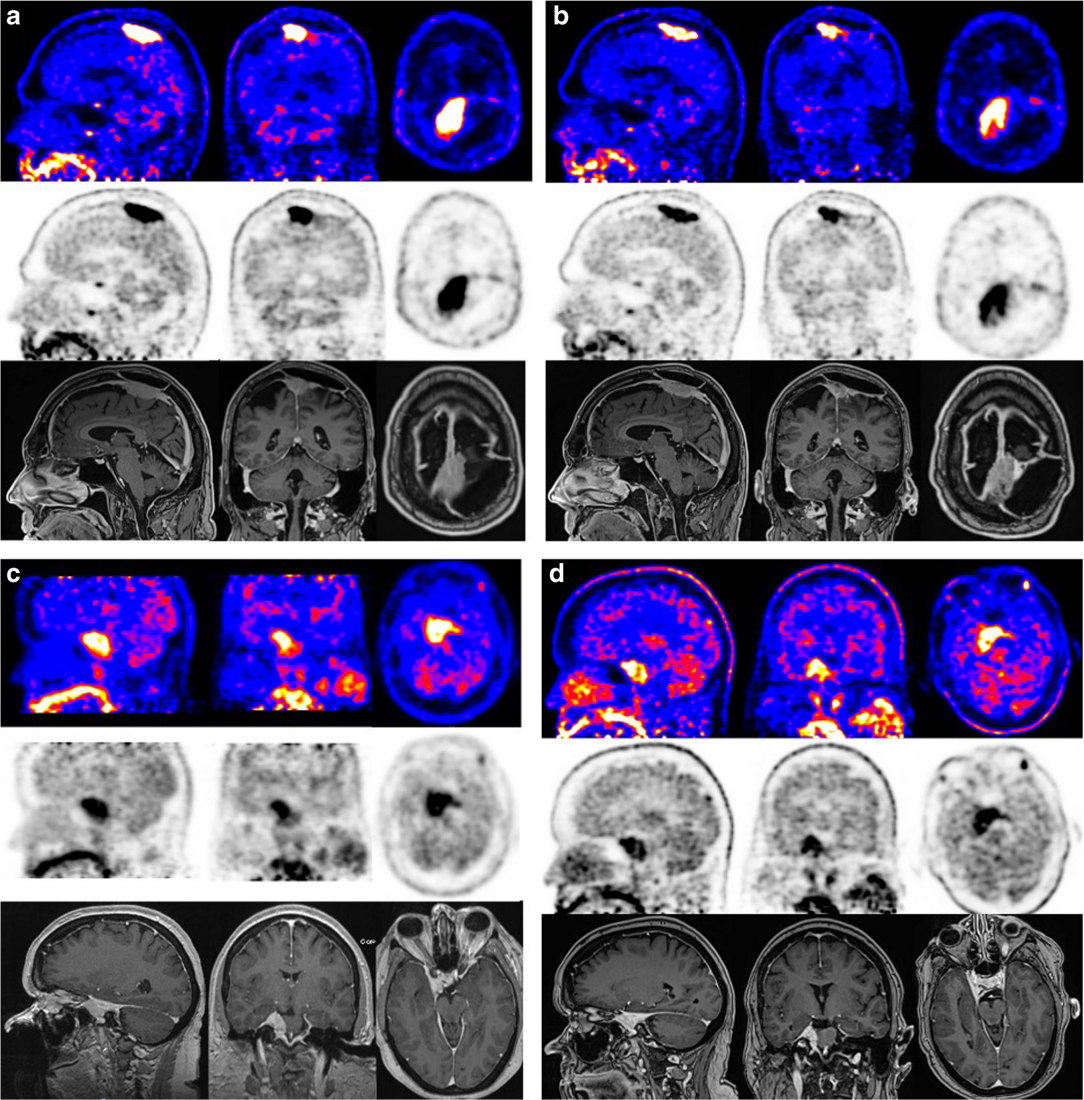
a Pre-SRT MET-PET and gadolinium-enhanced T1 MRI in a 62-year-old male patient, with previous partial resection of a parasagittal meningioma WHO grade I in September 2013. There is infiltration in the superior sagittal sinus. Because of growth of the tumor remnant during follow-up, SRT was installed in October 2017. b Post-SRT MET-PET and gadolinium-enhanced T1 MRI in the same, now 63-year-old patient, 15 months after SRT. MET-PET shows persistent raised ^11^C-methionine uptake in the tumor region. c Pre-SRT MET-PET and gadolinium-enhanced T1 MRI in a 63-year-old female patient with a meningioma extending in the right cavernous sinus. In December 2011, SRT was installed, without prior surgical resection. The treatment plan was made based on pathognomonic radiologic imaging. No tumor biopsy was performed. d Post-SRT MET-PET and gadolinium-enhanced T1 MRI in the same, now 70-year-old patient, 84 months after SRT. MET-PET shows persistent raised ^11^C-methionine uptake in the tumor region
